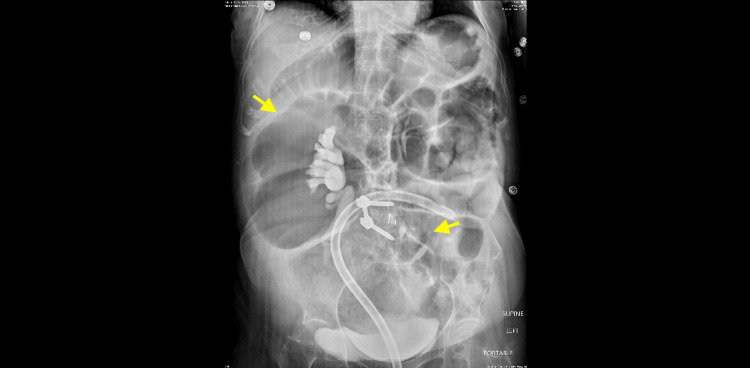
X-ray of the abdomen showing massive amount of stool in the sigmoid colon and rectum, reflecting impaction (yellow arrows). The rectal tube tip projects over the left iliac crest.
